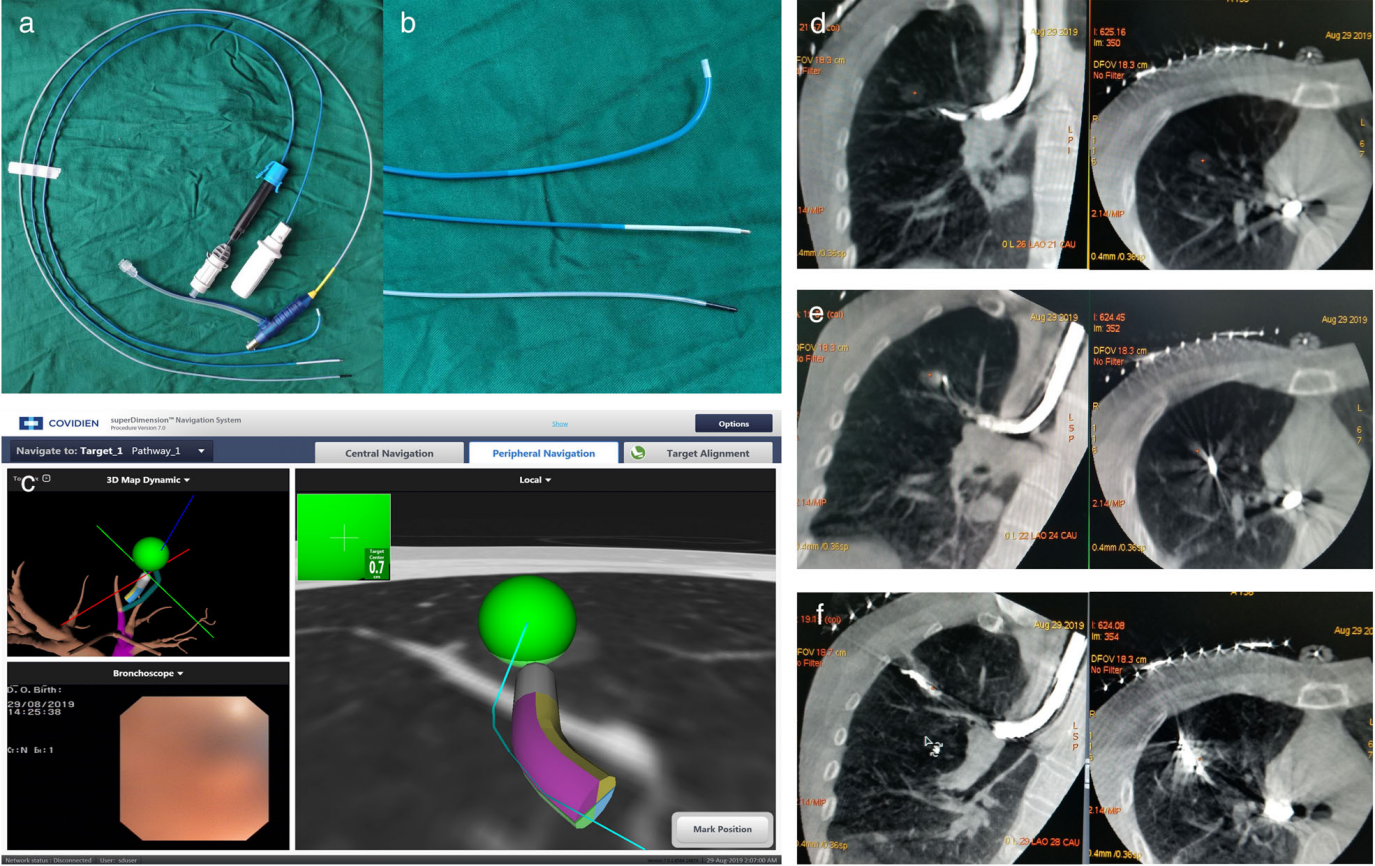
(a) The devices used for microwave ablation (MWA) guided by electromagnetic navigation bronchoscopy (ENB) including the ENB working channel catheter (curved‐tip catheter), magnetic probe and customized microwave antenna (with black short needle head), respectively. (b) Magnified image of the three devices. (c) ENB image shows that the working channel catheter has accurately reached a position adjacent to the lesion by guidance of the magnetic probe. MWA guided by ENB in CBCT image. (d) The magnetic probe and working channel catheter were inserted into the bronchus through the bronchoscope. (e) CT image shows that the antenna arrived at the lesion through the working channel catheter before MWA commenced. (f) After MWA was guided by ENB, the area and density of the shadow increased in the location of the lesion.
